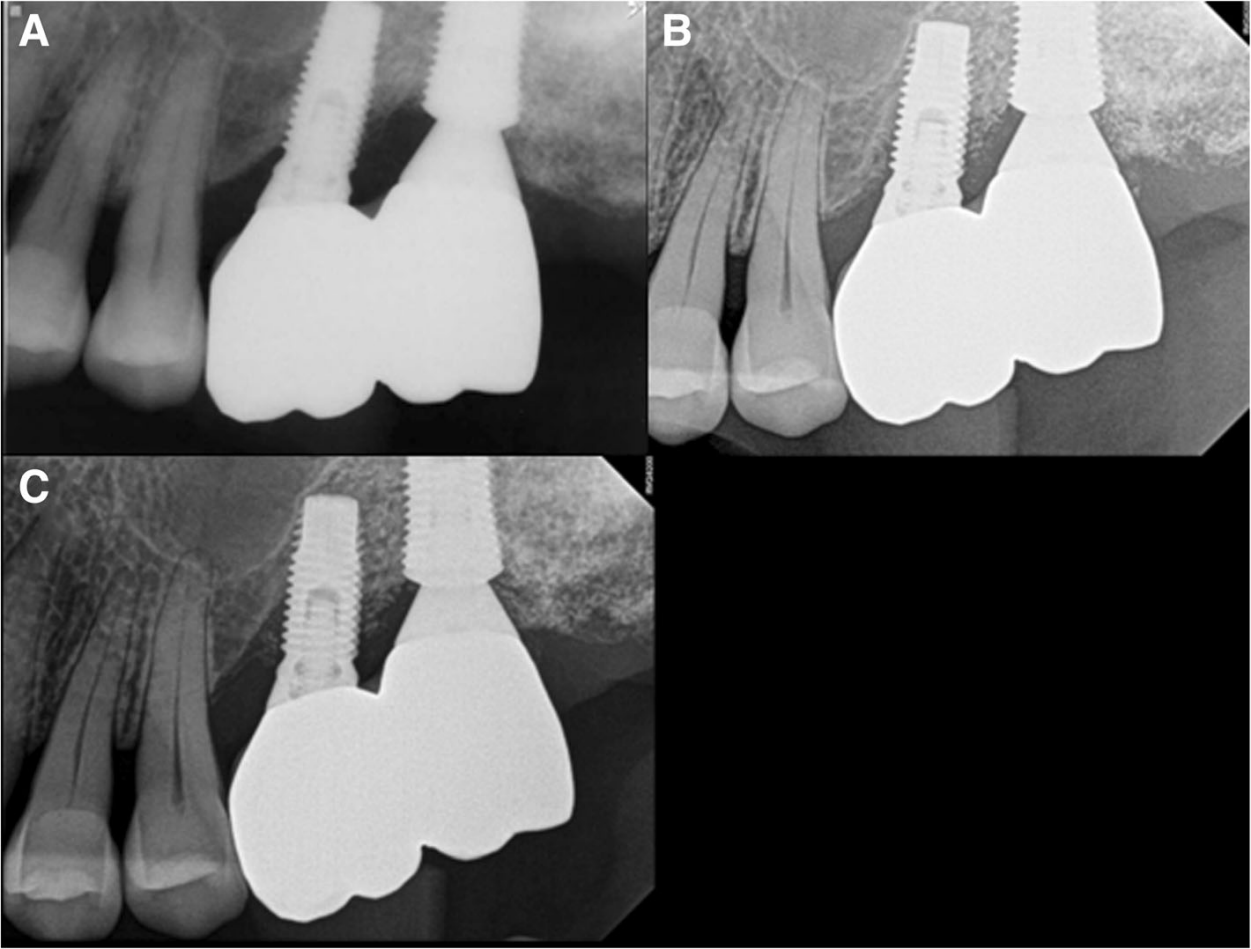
F2, periapical radiograph, a #27i, prosthesis delivery, b #27i peri-implantitis, #26i increasing radiolucent lesion, c #27i peri-implantitis, #26i mesial bone loss
